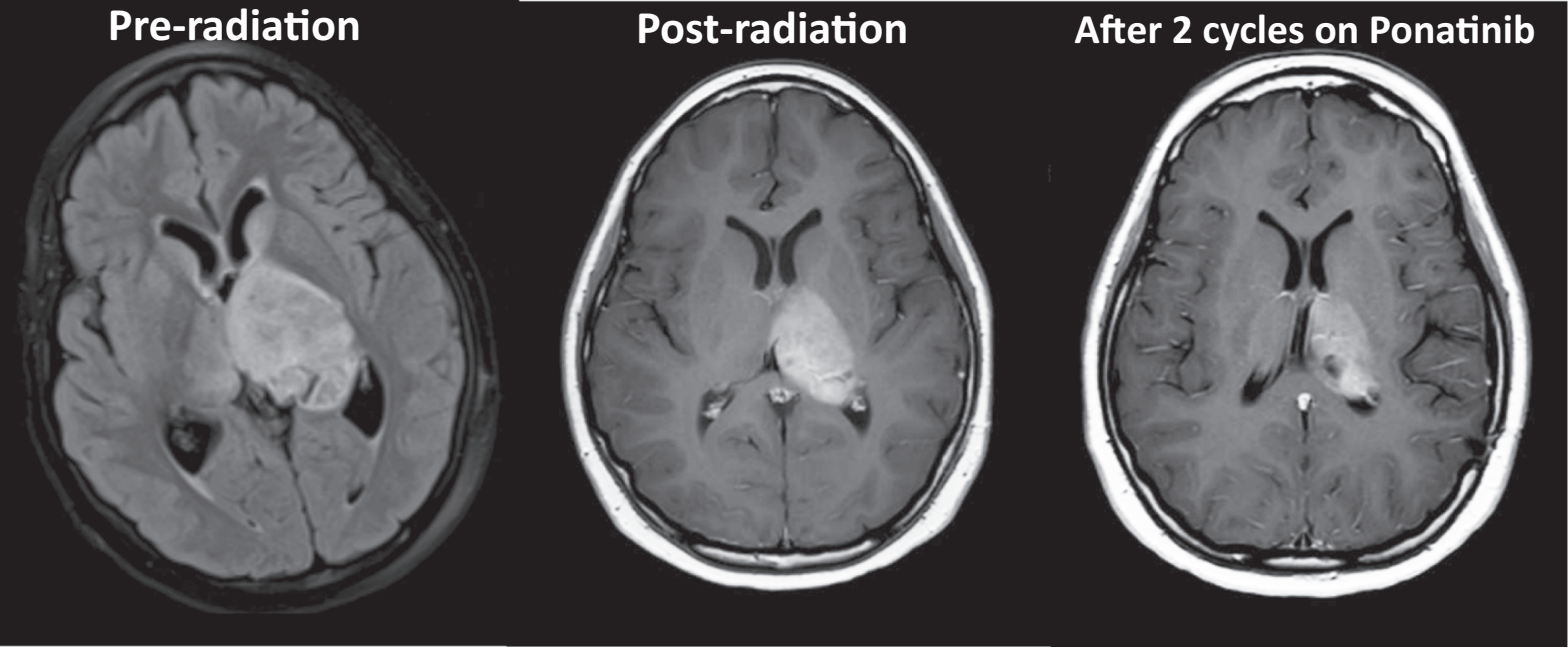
Axial T2 FLAIR image of the thalamic tumor pre-radiation (left), post-radiation (November 2016, center) and after two cycles of ponatinib (March 2017, right). Dimensions of the post-radiation tumor are 45.6 mm × 28.6 mm × 35.0 mm compared to 40.3 mm × 26.6 mm 36.0 mm after two cycles on ponatinib. This represents a 15% reduction in volume
